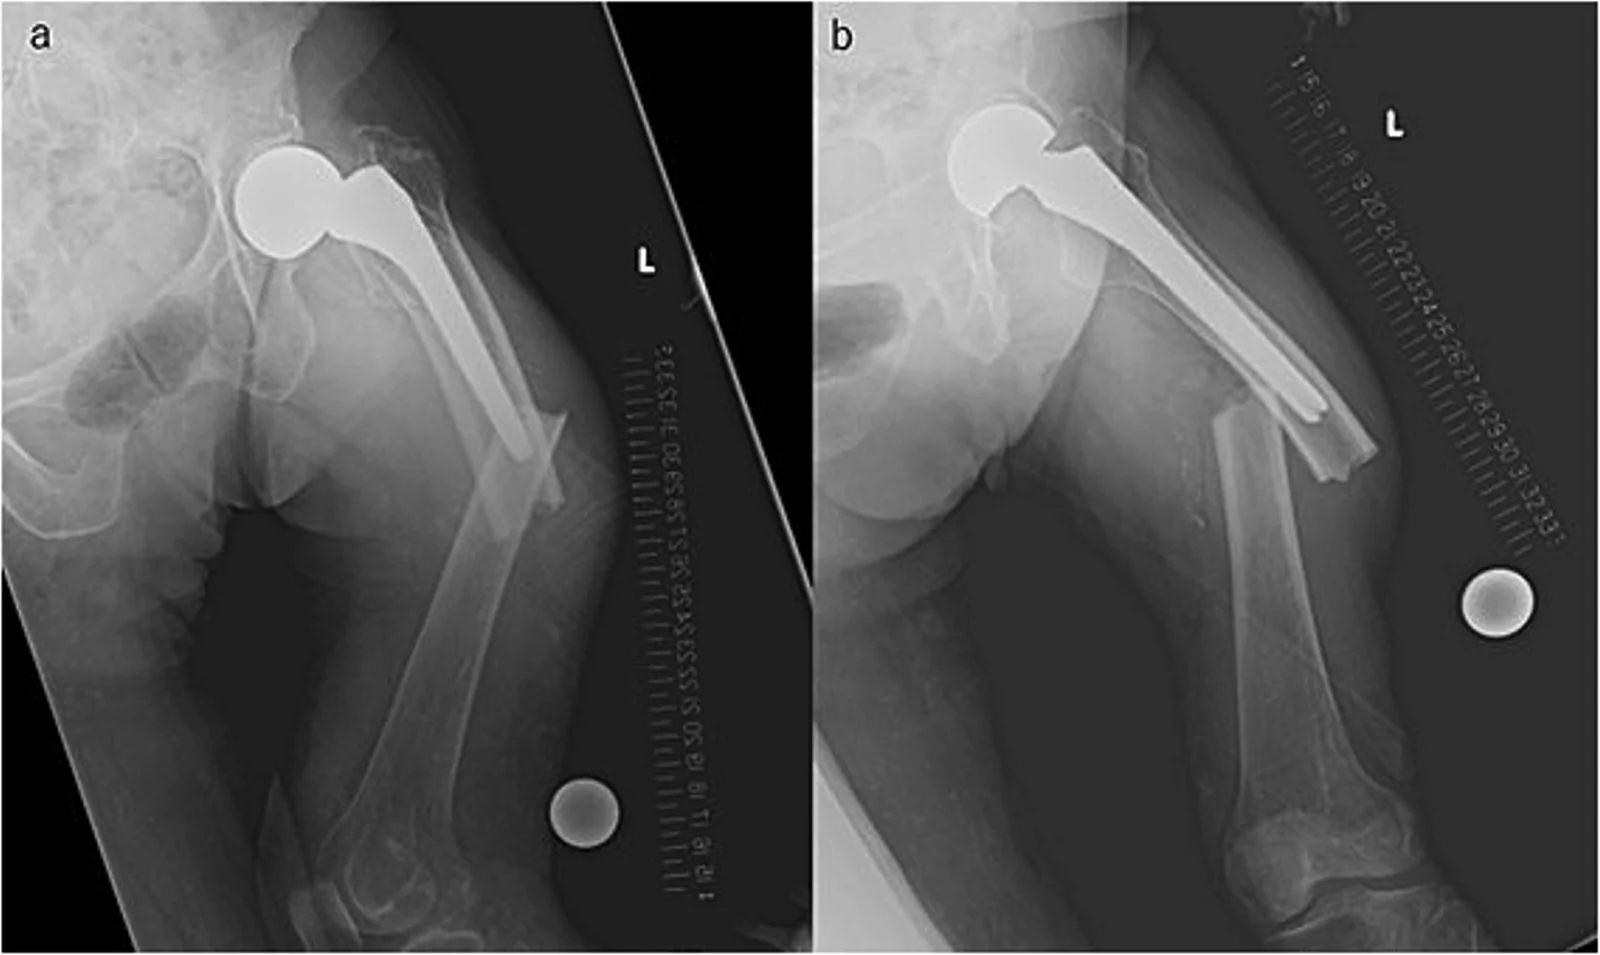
Anteroposterior (a) and lateral (b) plain radiographs obtained at presentation. A transverse, non-comminuted fracture with lateral cortical thickening and femoral bowing is demonstrated, consistent with the radiographic appearance of an atypical femoral fracture.
